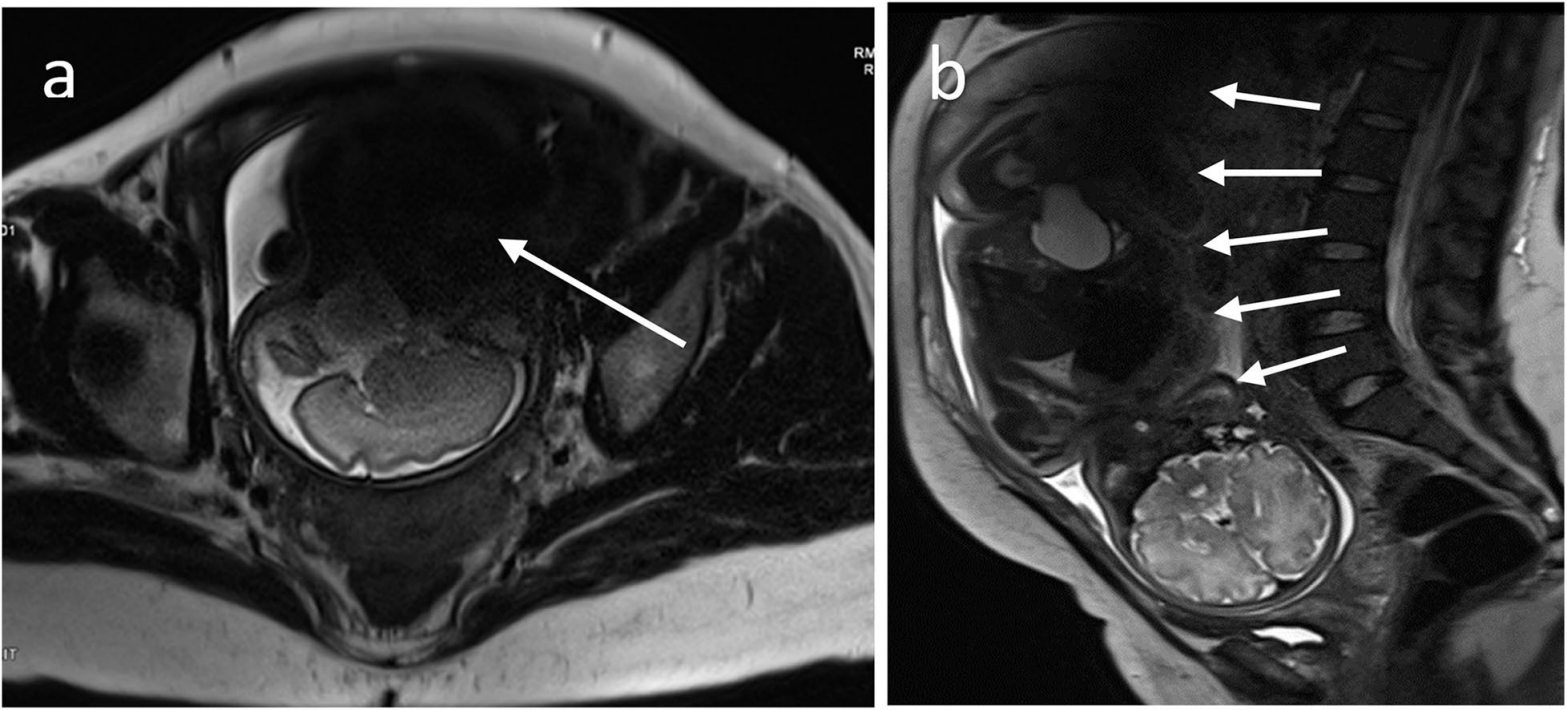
Sagittal and coronal views of a 34-week fetus acquired with a T2-weighted single-shot fast spin-echo sequence: sagittal plane of fetal brain corrupted by unpredicted fetal motion (arrow) (a). Coronal view of a fetal body corrupted by dielectric artifact (arrows) (b)
